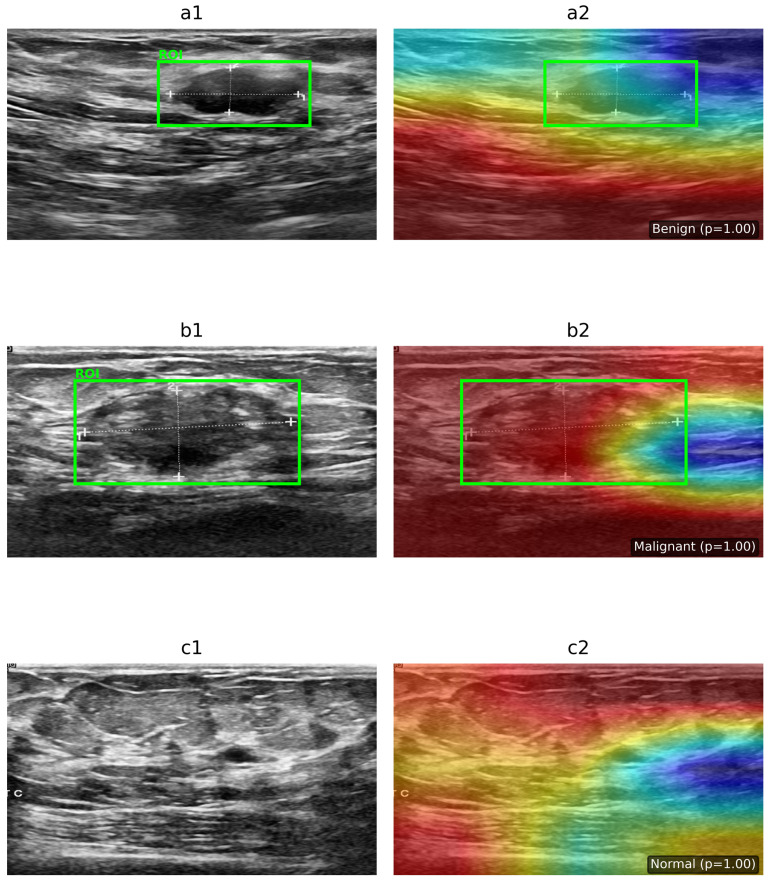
Interpretability analysis using Vision Transformer (ViT) attention maps on BUSI ultrasound images. The left column shows the original ultrasound images with radiologist-defined regions of interest (ROI): (a1) benign, (b1) malignant, and (c1) normal cases. The right column shows the corresponding ViT attention maps: (a2) benign, (b2) malignant, and (c2) normal. The model exhibits localized attention in lesion regions for benign and malignant cases, while the normal case shows more diffuse attention patterns.
